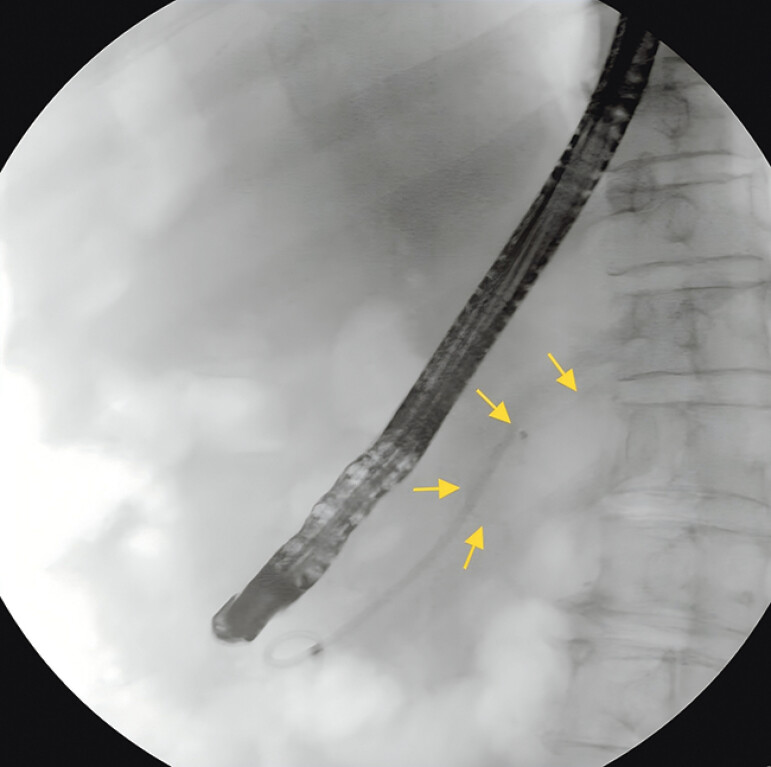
Radiography indicated that the stent was displaced into the pancreatic duct.
